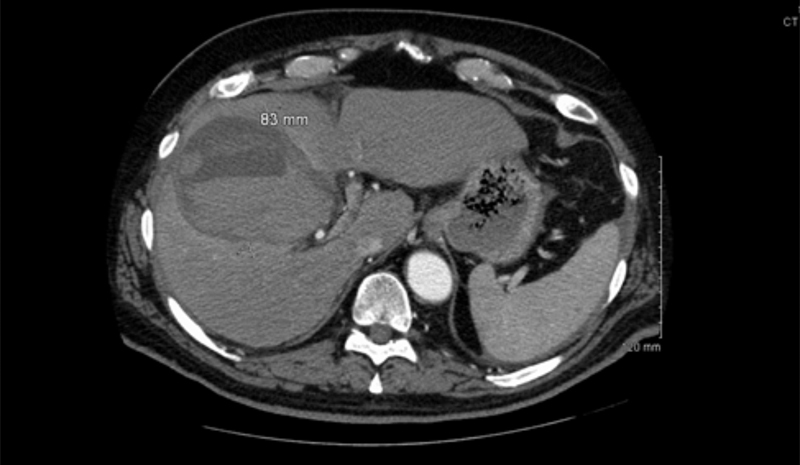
Computed tomography (CT) of abdomen and pelvis showing distended hemorrhagic appearance of gallbladder, no stones.
